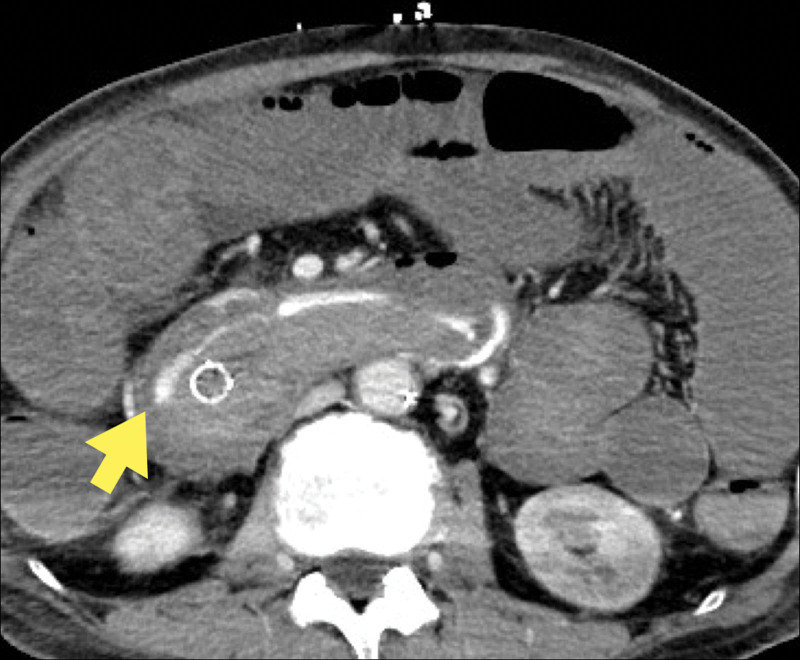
Contrast-enhanced CT scan showing extravasation from the PSPDA into the biliary tract. CT = computed tomography, PSPDA = posterior superior pancreaticoduodenal artery.
